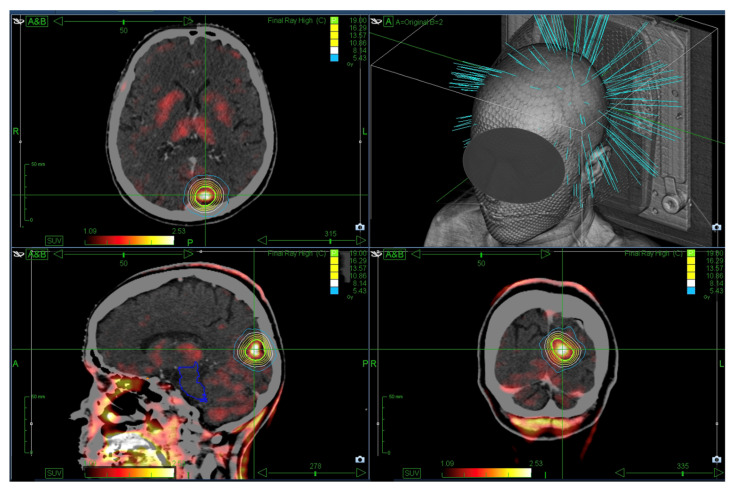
Exemplary single-session SRS plan showing a prescription dose of 1 × 19 Gy (green) enclosing the PTV. The planning CT was fused with FET-PET for target definition.
